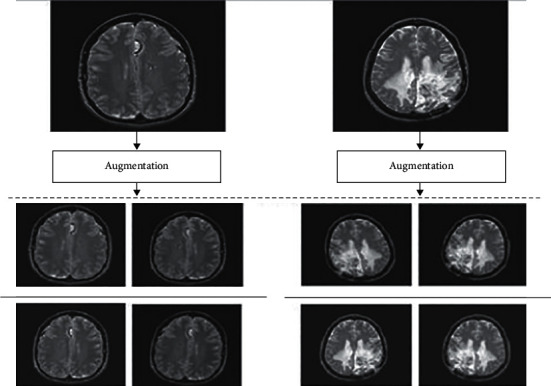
Augmented brain MRI image dataset.
